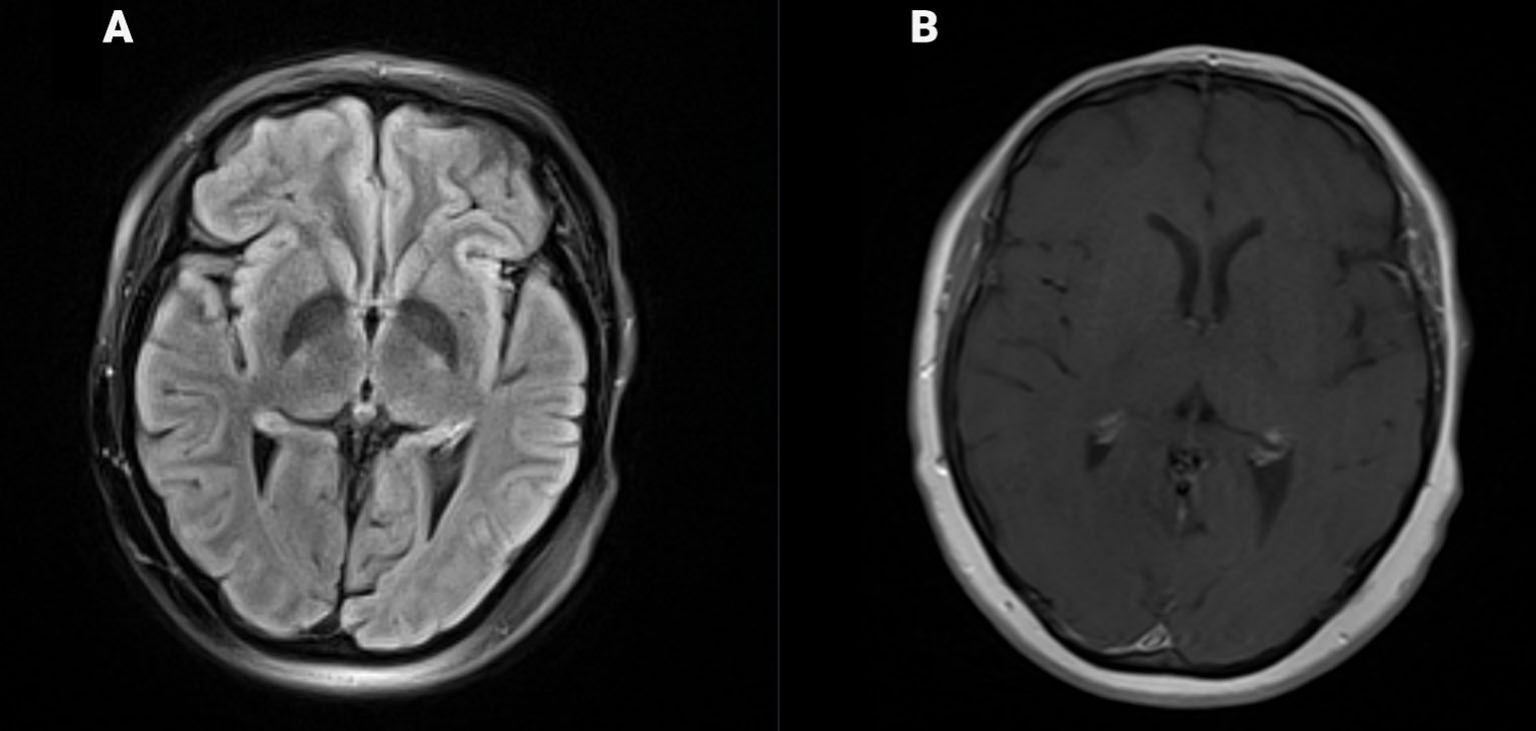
Axial MR image FLAIR demonstrates no T2/FLAIR signal intensity, suggesting the resolution of vasogenic edema in a previously seen region (A), with continued absence of enhancement within regions on axial MR brain T1 post-contrast images (B).
